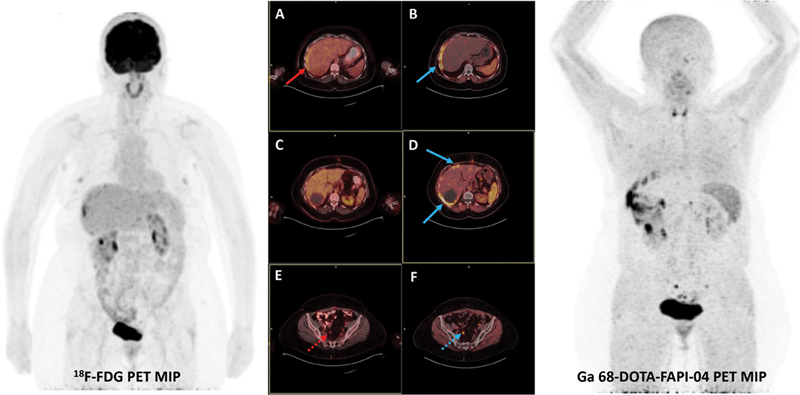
A 48-year-old woman in whom FAPI imaging demonstrated superior visualization of PC (FAPI SUVmax = 30.3, FDG SUVmax = 12.7). (
